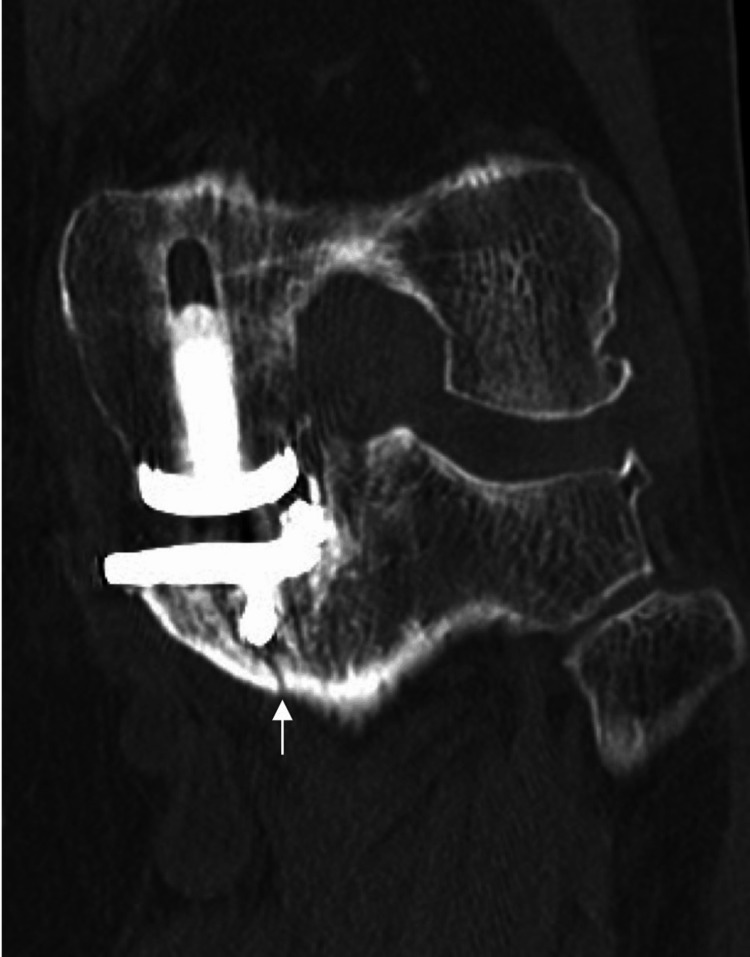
Four years postoperative computed tomography of the peri-implant fracture
